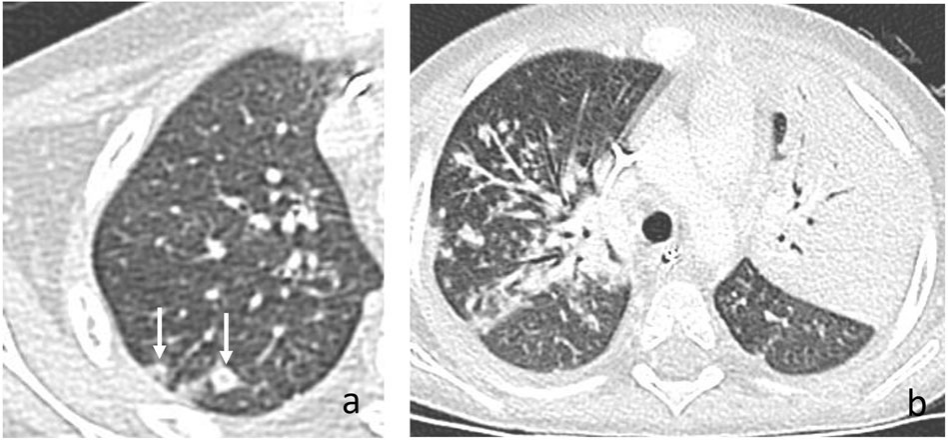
Proven pulmonary candidiasis in a 3-year-old boy with Burkitt’s lymphoma. The patient developed fever and increasing respiratory symptoms while in agranulocytosis under chemotherapy. Initial chest contrast-enhanced computed tomography (CE-CT) images on lung window (a) show a “reverse halo sign” in the right upper lobe (arrows). Follow-up CE-CT exam (b) one week later revealed rapid progression of the illness with multiple, varied-sized pulmonary nodules. A new extensive alveolar consolidation is detected in the left upper lobe
